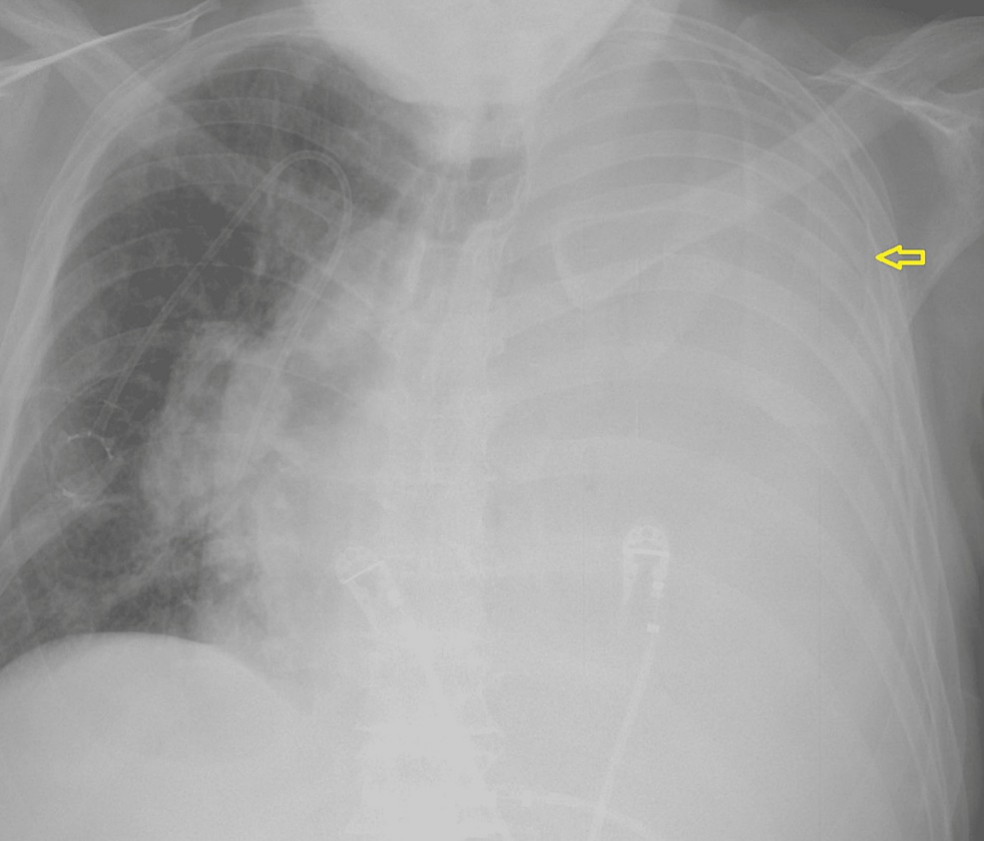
Chest radiograph demonstrating opacification of the entire left hemithroax
